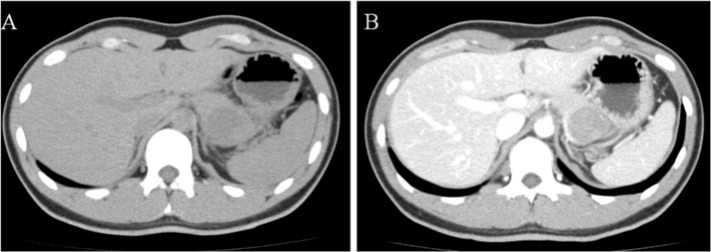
Abdominal computed tomography showing the solid pseudopapillary neoplasm in our patient. (A) This is a plain image, and it shows a mass of 40mm diameter in the pancreatic tail. (B) This is a contrast-enhanced image, and it shows an increasingly enhanced mass of 40mm diameter in the pancreatic tail.
